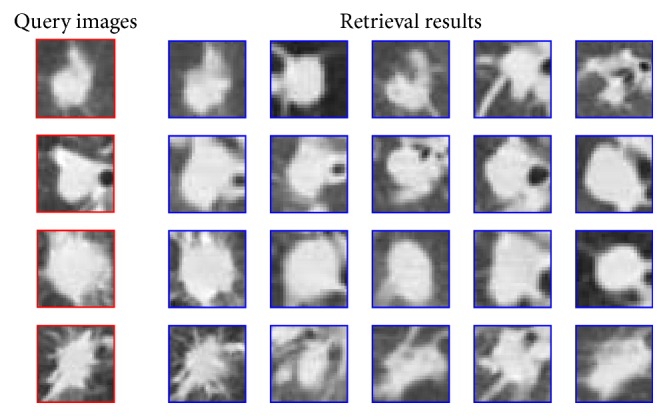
Retrieval results for lung nodule CT images using the proposed method.
